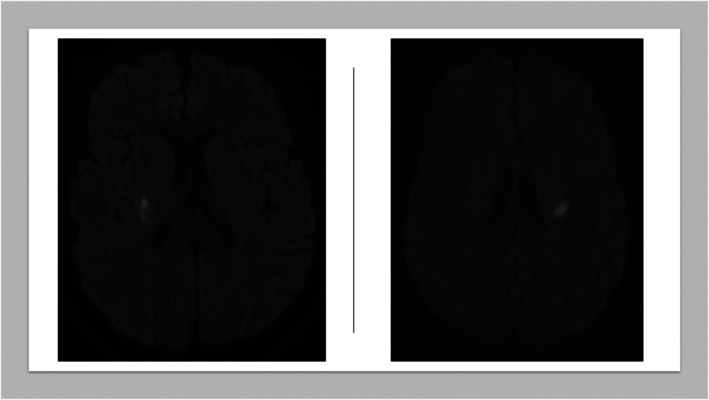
Two representative diffusion weighted MRI images of minor stroke. Note the small size and lack of involvement of eloquent cortex.
